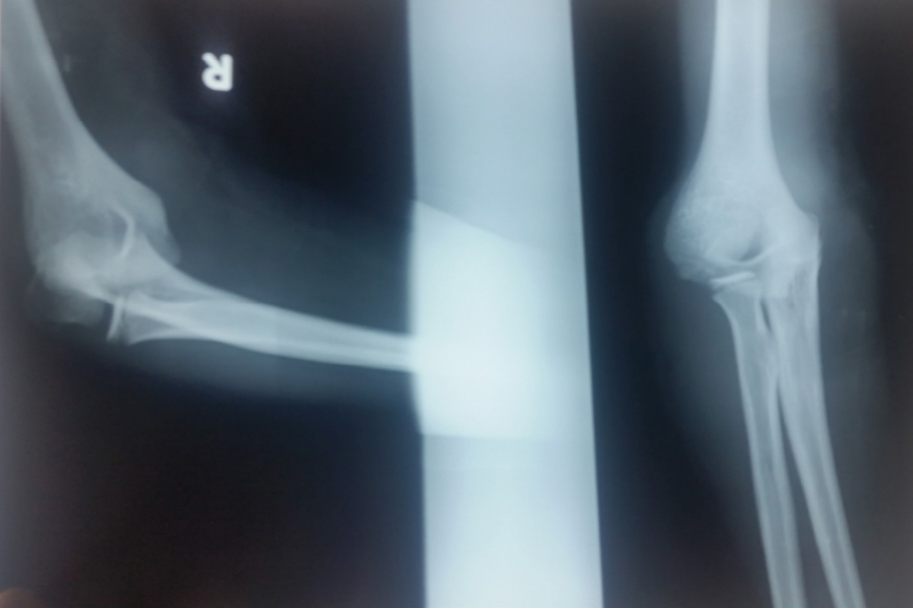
X-ray of right elbow isolated showing medial dislocation of right elbow.
